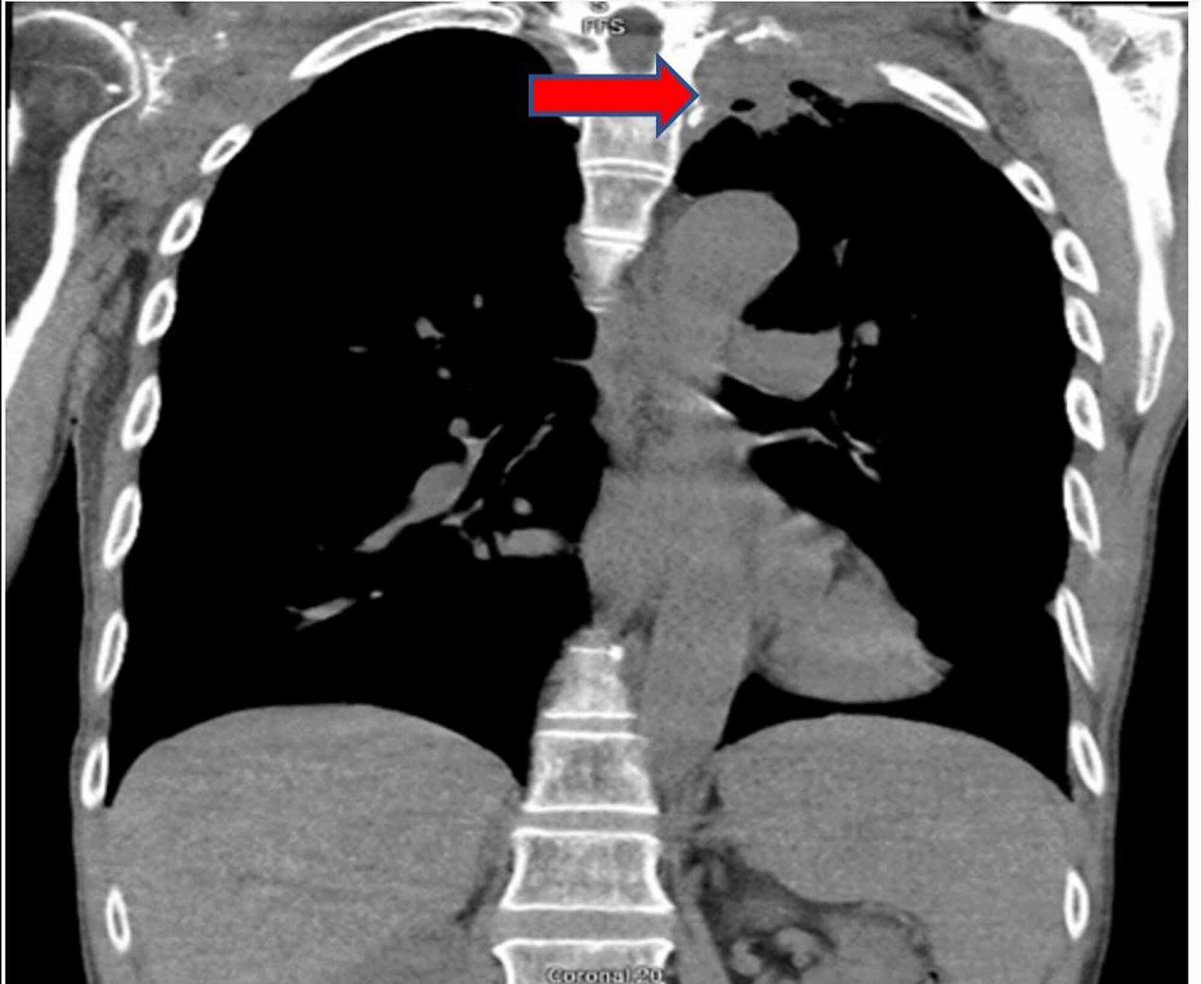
Coronal section CT chest. Red arrow showing mass on left side.
